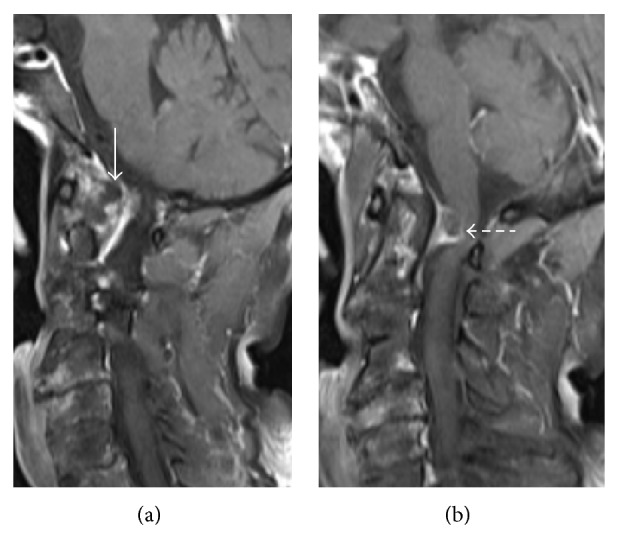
Postcontrast sagittal T1-weighted MR images reveal atlantoaxial tuberculosis with peripherally enhancing epidural abscess (*arrow*) accompanied by cord compression (*dashed arrow*) in a 62-year-old female who presented with gradual bilateral paralysis.
